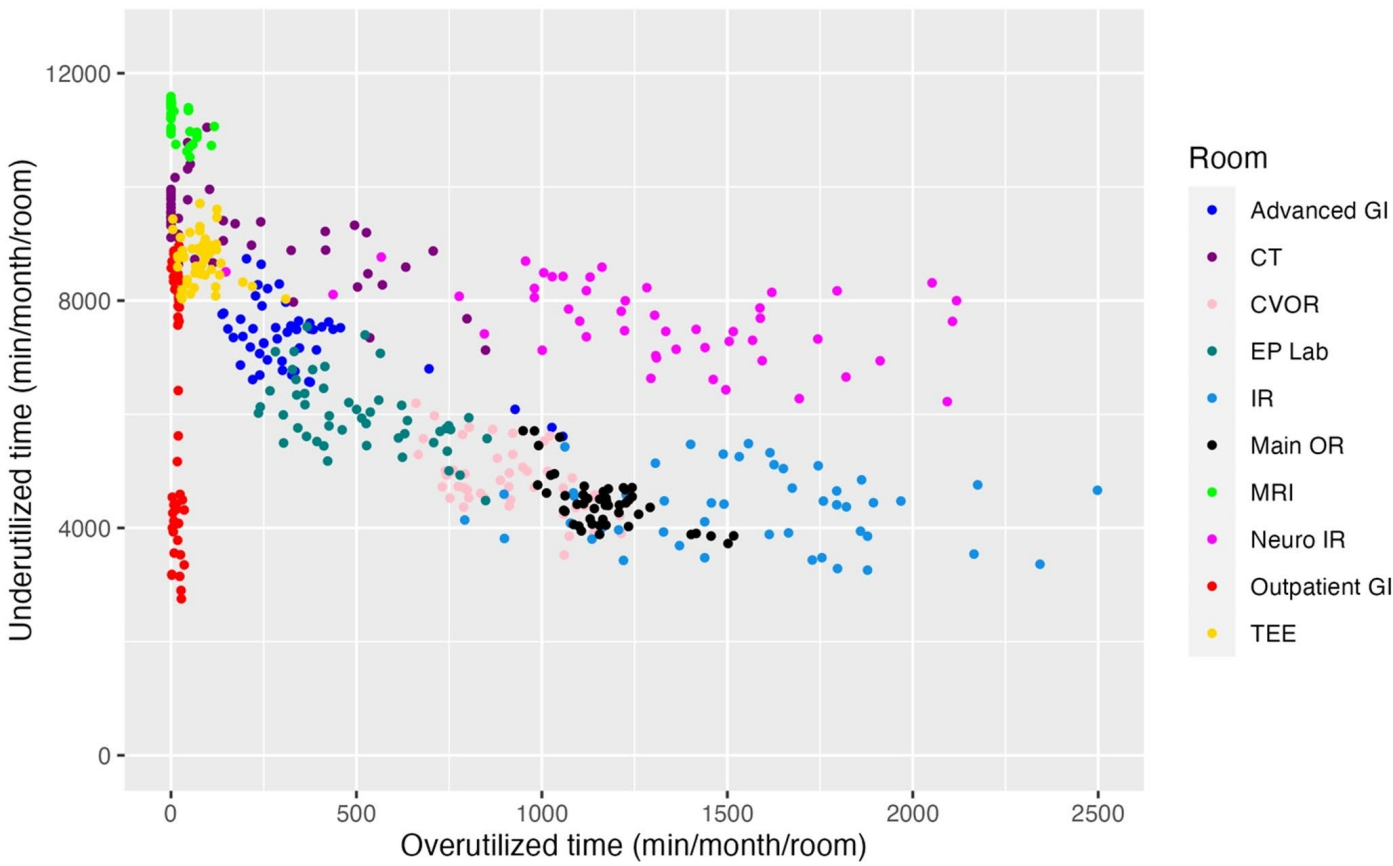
Under- and over-utilized minutes for various operating room and non-operating room anesthesia locations using normalized 4-week rolling sums on a per room basis. CT, computed tomography; CVOR, cardiovascular operating rooms; EP, electrophysiology; GI, gastroenterology; IR, interventional radiology; MRI, magnetic resonance imaging; OR, main operating rooms; TEE, transesophageal echocardiography
